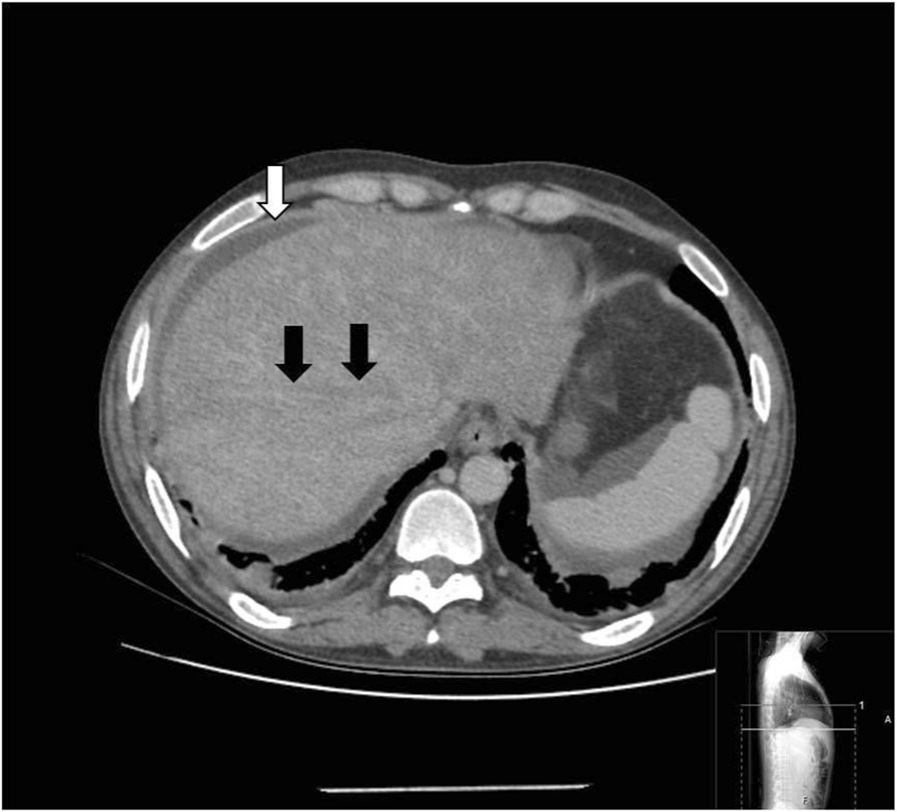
In axial venous phase contrast CT section; Mosaic pattern compatible with Budd-Chiari Syndrome is observed in the liver parenchyma. No contrast agent filling is observed in the hepatic veins (black arrows). In addition, acid is observed in the perihepatic area (white arrow)
